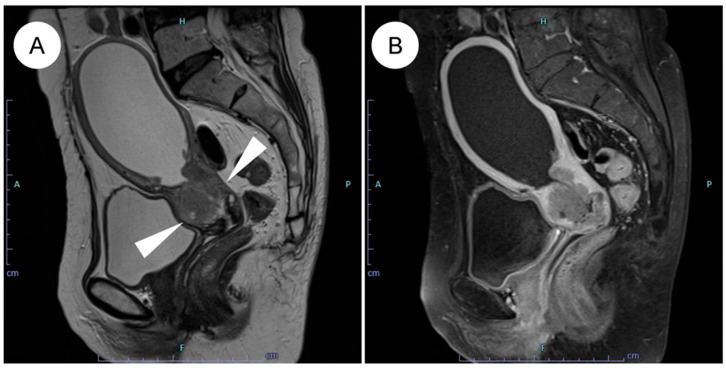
MRI features of HPV-independent gastric-type endocervical adenocarcinoma. (A) On sagittal T2-weighted imaging, an endophytic, mildly hyperintense mass is seen in the upper cervix (white arrowheads), showing diffuse infiltrative growth with small intratumoral cysts and associated hydrometra. (B) On post-contrast T1-weighted imaging, the tumor demonstrates mild enhancement. Note the mild enhancement compared to the typically more avid enhancement seen in usual-type adenocarcinoma.
